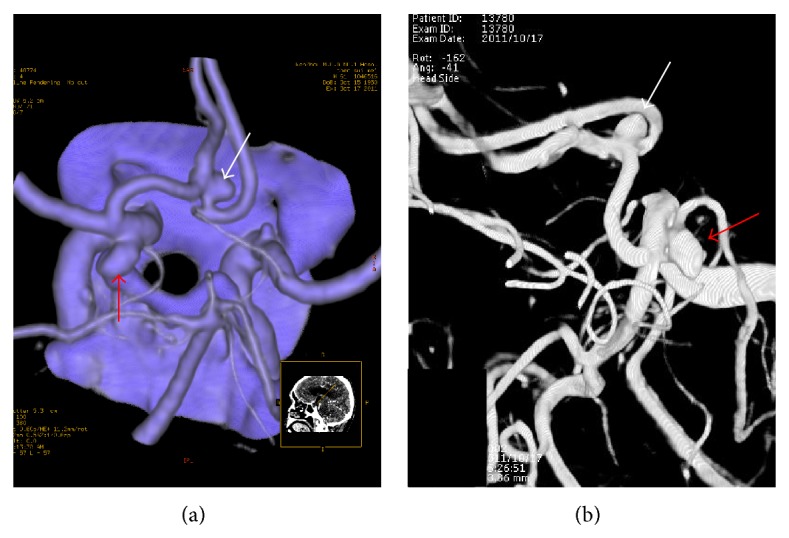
Images from a 61-year-old woman with SAH. L-CTA scanning shows an aneurysm ((a), white arrow) at anterior communicating artery and an aneurysm ((a), red arrow) at posterior communicating artery. 3D-DSA depicts the aneurysms at the same locations ((b), white and red arrows). The maximum diameter and neck sizes of anterior communicating artery aneurysm were 7.32 mm and 3.40 mm ((b), white arrow); and those of posterior communicating artery aneurysm were 5.44 mm and 3.86 mm ((b), red arrow).
